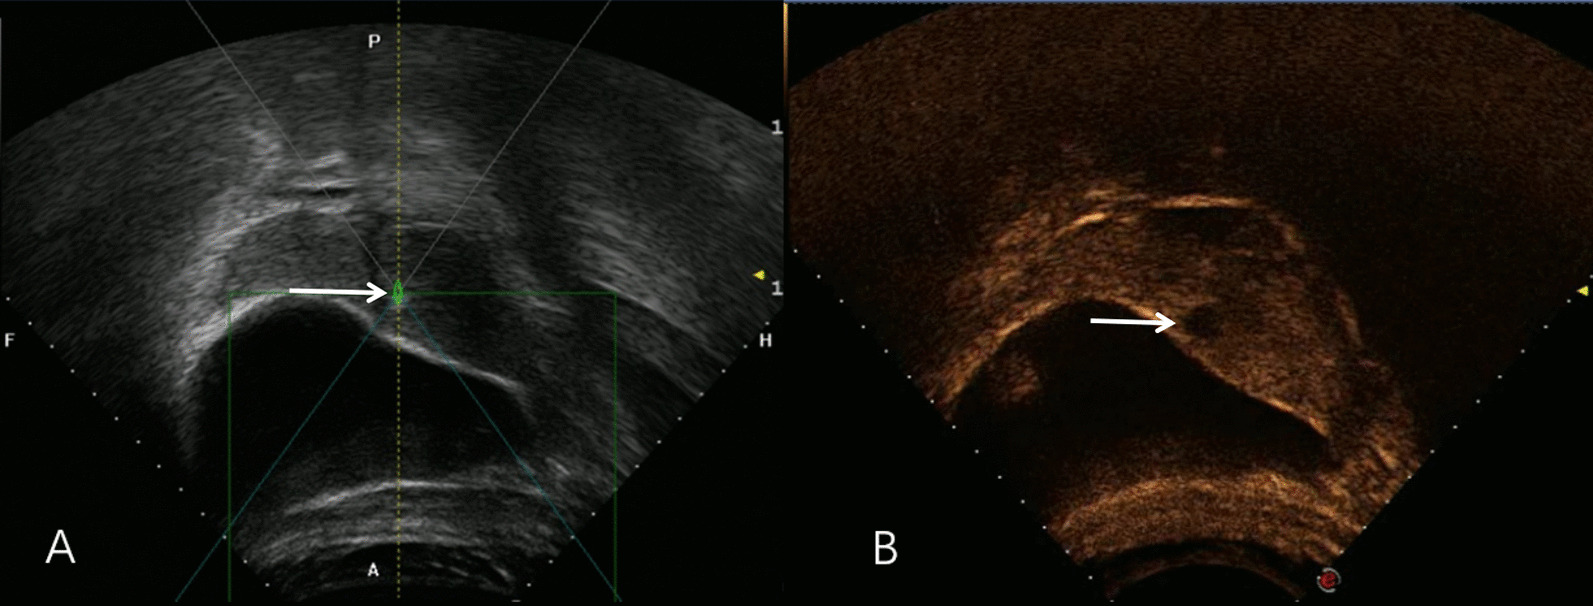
Ultrasound-guided HIFU treatment. Lumpy gray-scale change was seen during the surgery (A), while the vascular flow disappeared and the contrast-enhanced ultrasound showed no perfusion of uterine lesion immediately after the surgery (B)
